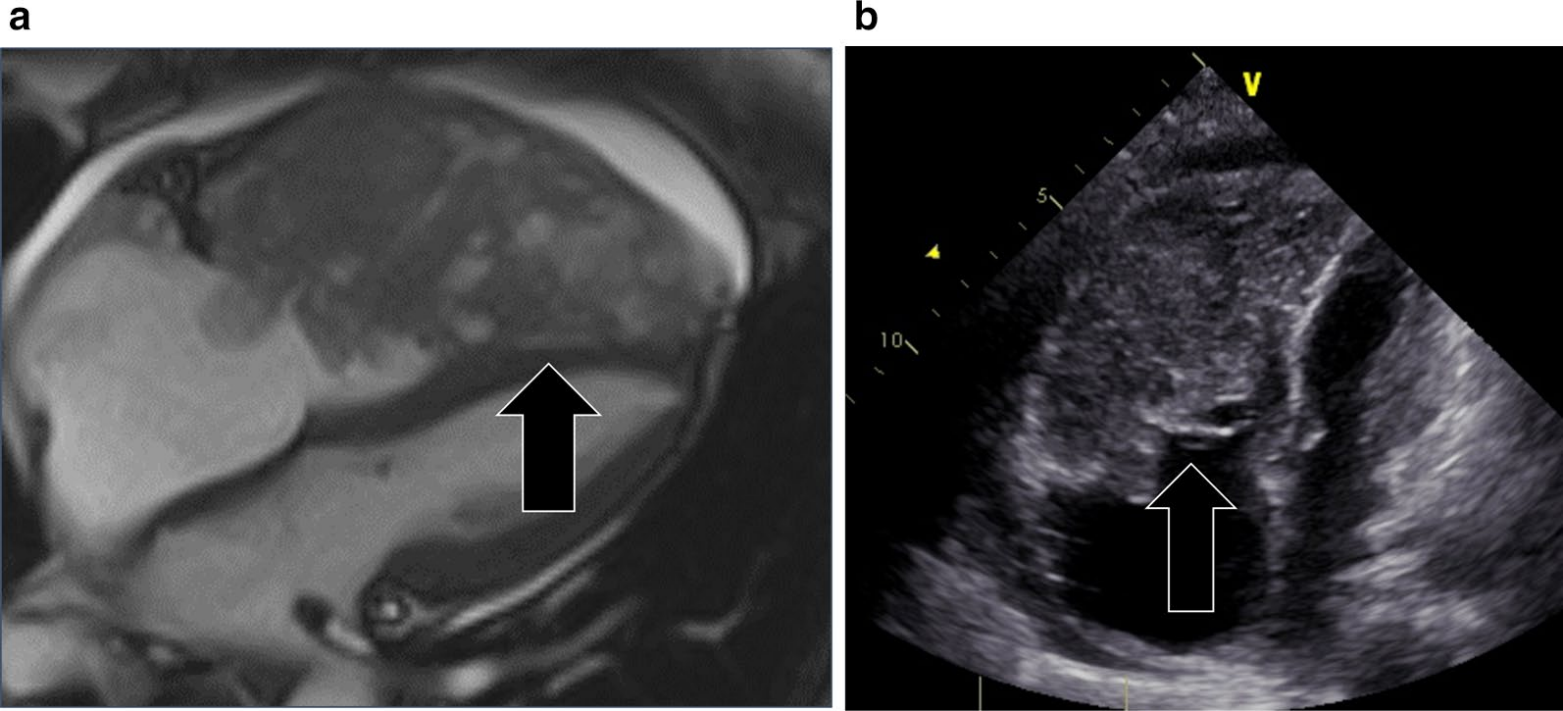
Image at last follow-up. Magnetic resonance imaging (a) and echocardiography (b) confirm a massive metastasis in the right ventricle (arrow)
